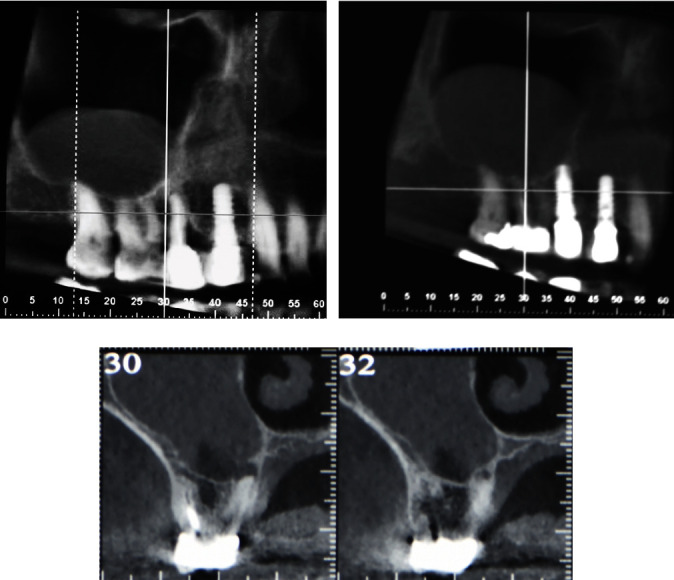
(a, b, and c) CBCT pre surgery showing the right first upper molar with a palatal radiopaque lesion associated with a cupoliform lesion inside the maxillary sinus.
